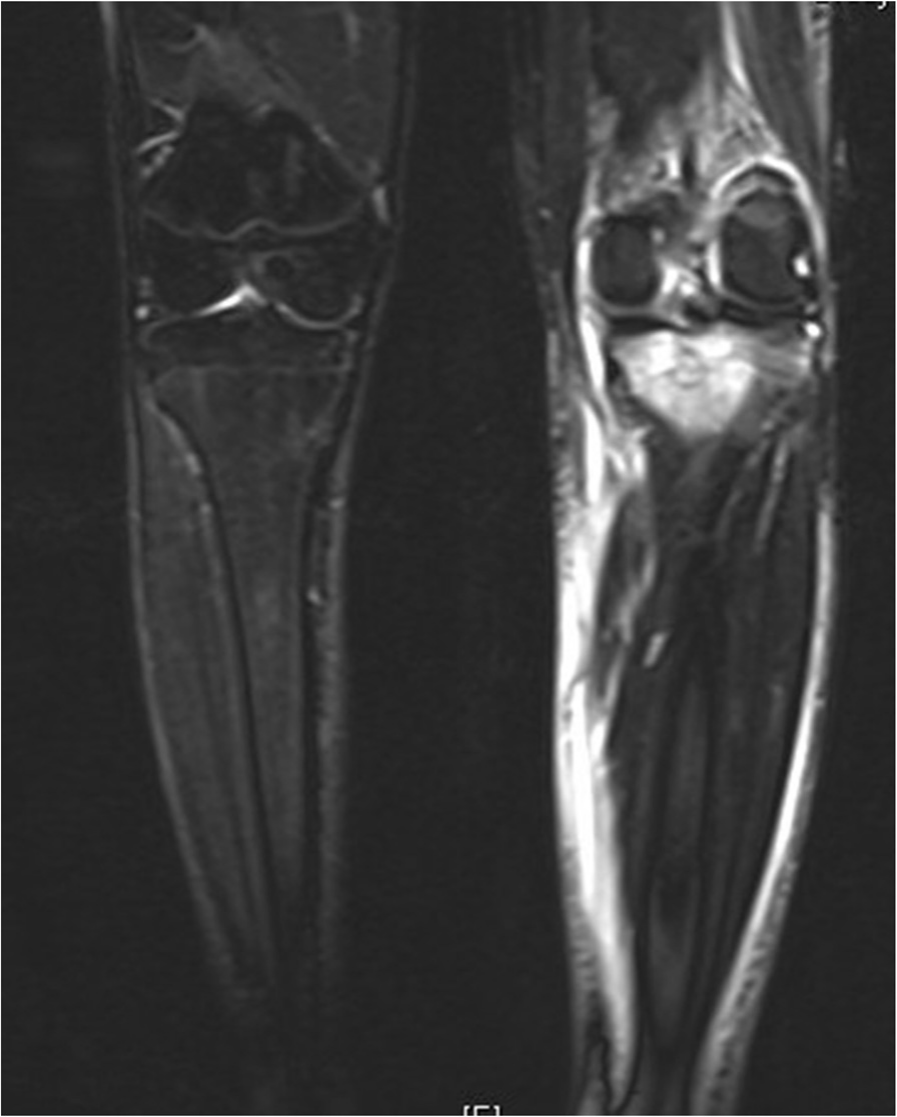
Magnetic resonance imaging scan of the left knee, taken at the day of the acute presentation, showing the leg fasciitis and myositis
